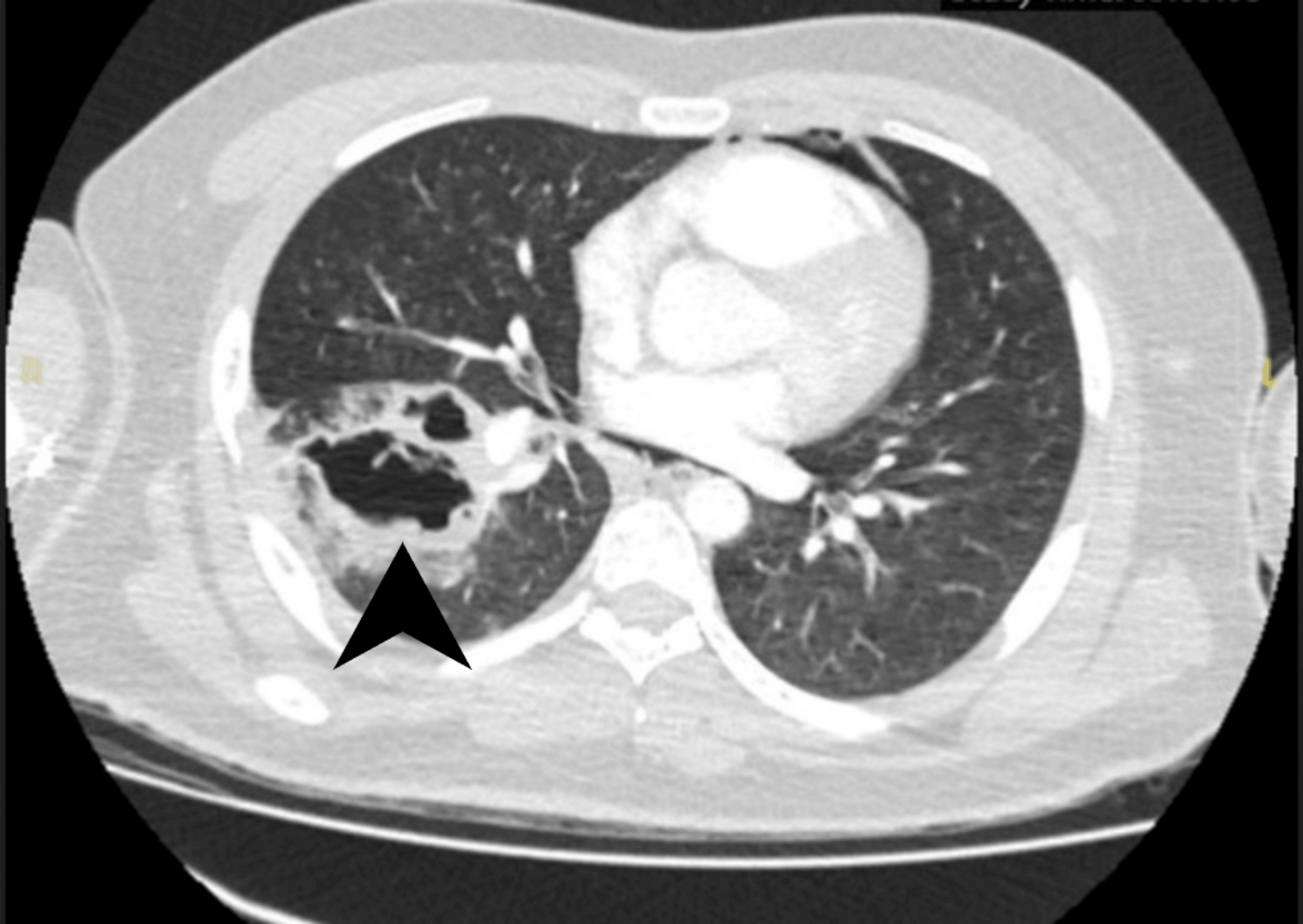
Axial contrast-enhanced CT of the thorax showing cavitating pneumonia in the right lower lobe with associated pneumomediastinum. Arrowhead points to the cavitary lesion, with surrounding features of pneumomediastinum. CT: computed tomography
